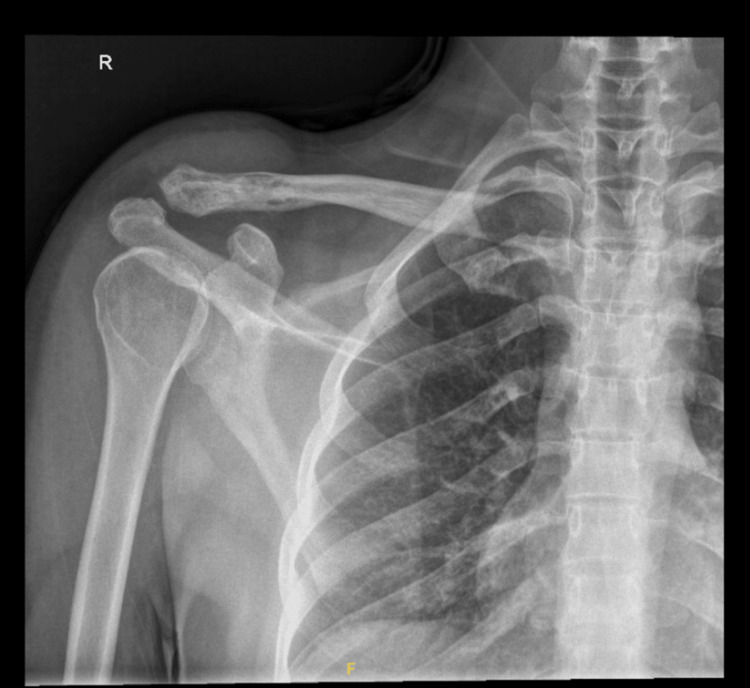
Preoperative X-ray of the patient's right shoulder
